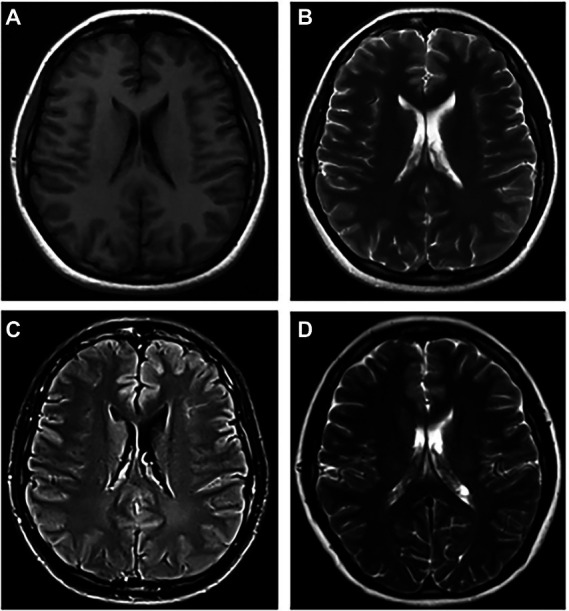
The abnormal MRI was obtained from patient 7. A 26-year-old woman with concomitant CSF MOG and NMDAR antibodies presenting on admission with severe headache, low-to-moderate fever, vision loss, and Limb jitter. Lumbar puncture show a significant increase in intracranial pressure, CSF pleocytosis and CSF protein elevation. Axial T1-weighted (A) and T2-weighted (B) images show normal in the left frontal lobe, but Flair image (C) appeared slight abnormal signal. After HIMP shock therapy, the patient leave the hospital with a decrease in laboratory indicators of CSF and no abnormalities on MRI (D).
